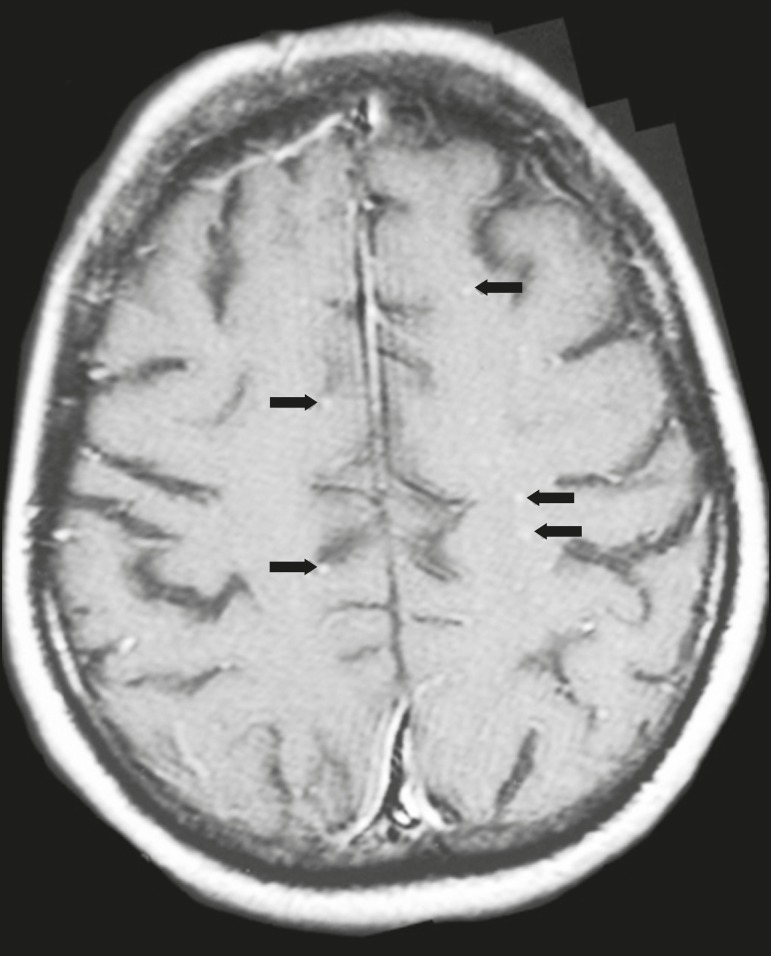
Case 5.* Contrast-enhanced T1-weighted image showing miliary
